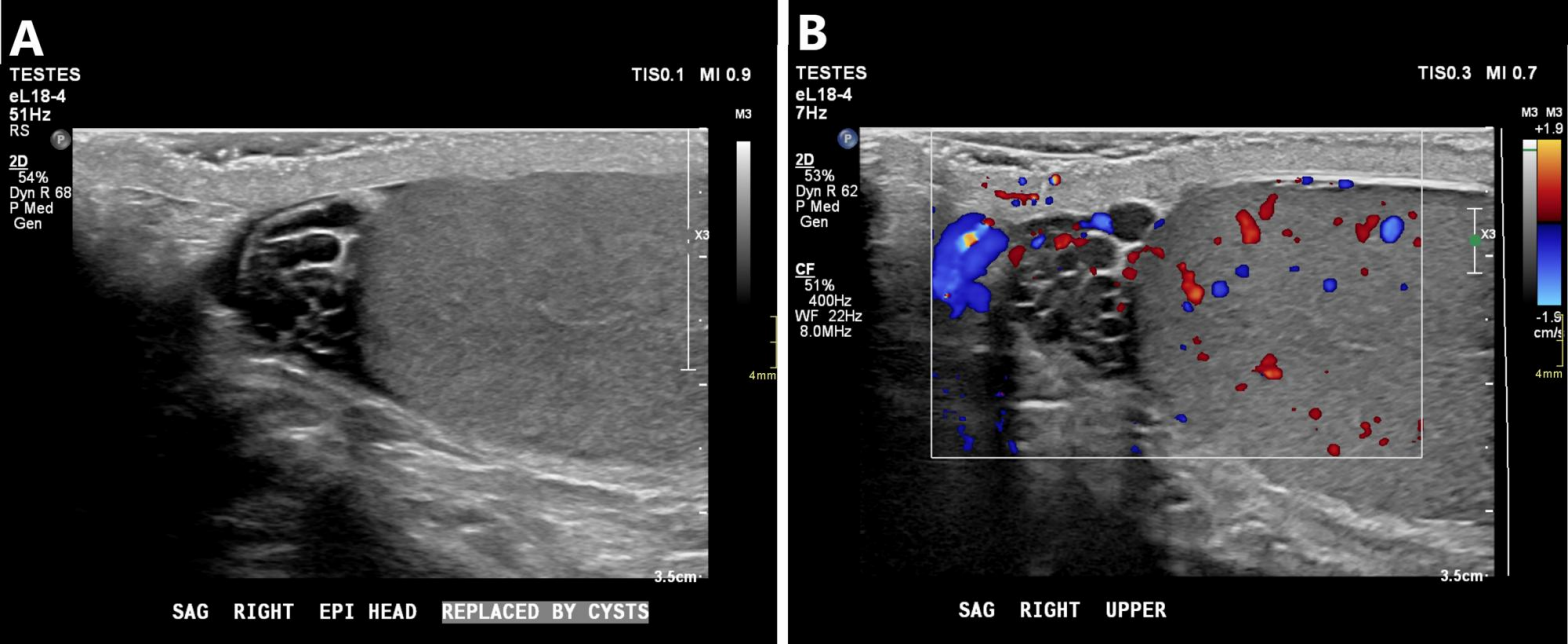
Spermatocele. (A) The epididymal head is nearly completely replaced by cystic lesions with echogenic debris. (B) Doppler interrogation proves that the cystic structures are not blood vessels.
